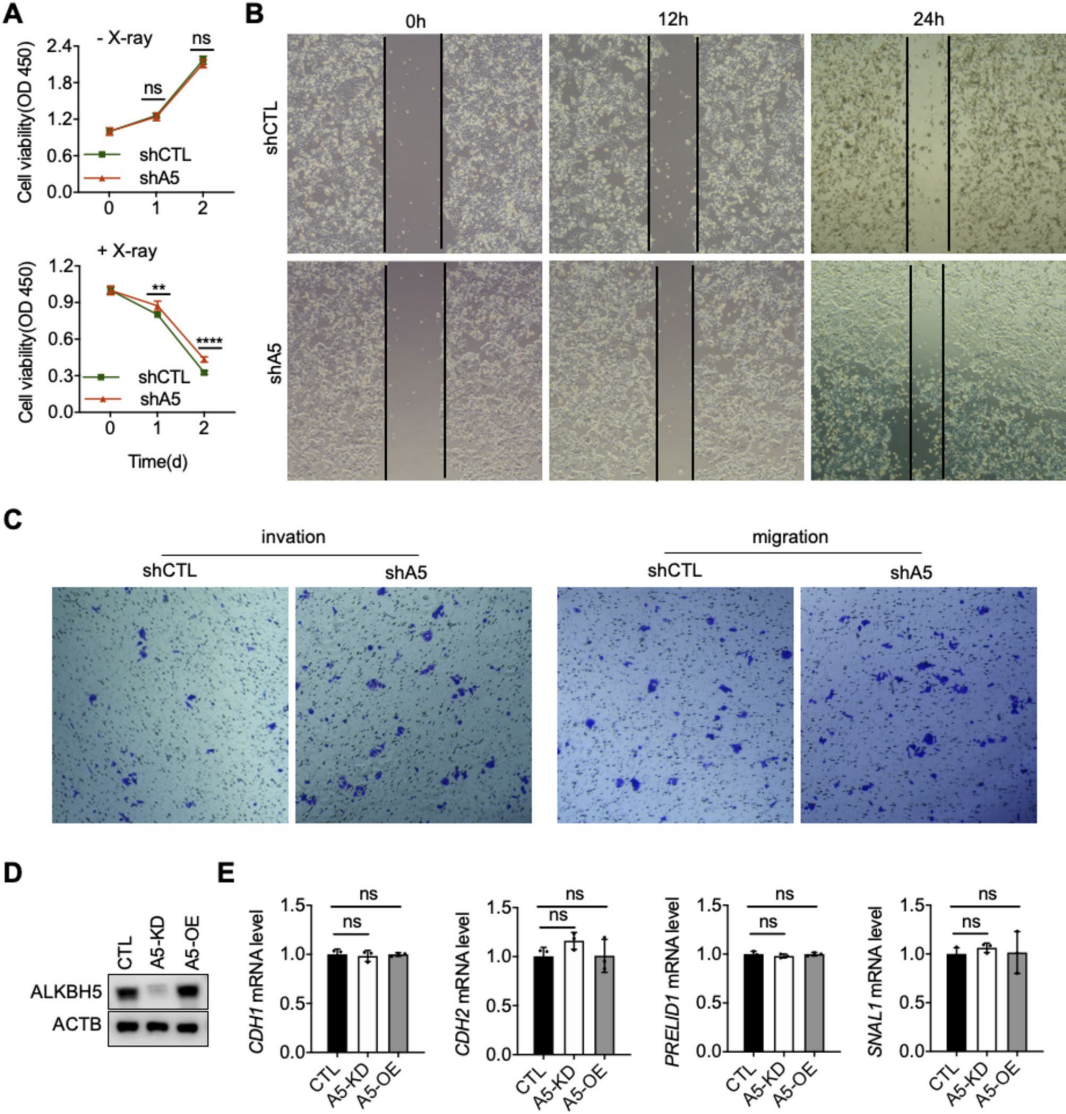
ALKBH5 promotes the malignant phenotype of HCT116 cells (10 Gy). (A) Cell viability of HCT116 cells with or without X-ray treatment (*n* = 5, *t*-test). (B) Representative image of wound healing assay of HCT116 cells. (C) Representative image of transwell assays of HCT116 cells. (D) Validation of A5 knockdown and overexpression. Original blots are presented separately. (E) The mRNA level of EMT markers in HCT116 cells following X-ray exposure. *P* < 0.01, P* < 0.001, *P* < 0.0001.
